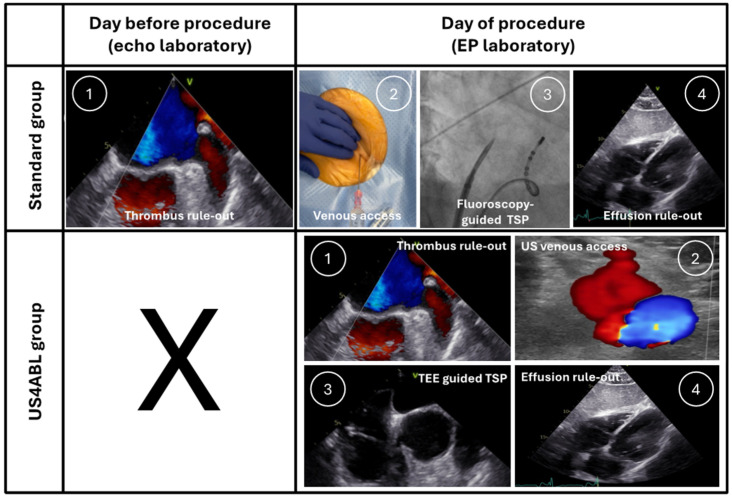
Comparison between the standard workflow and the US4ABL strategy. US—ultrasound; TEE—transesophageal echocardiography; TSP—transseptal puncture.
